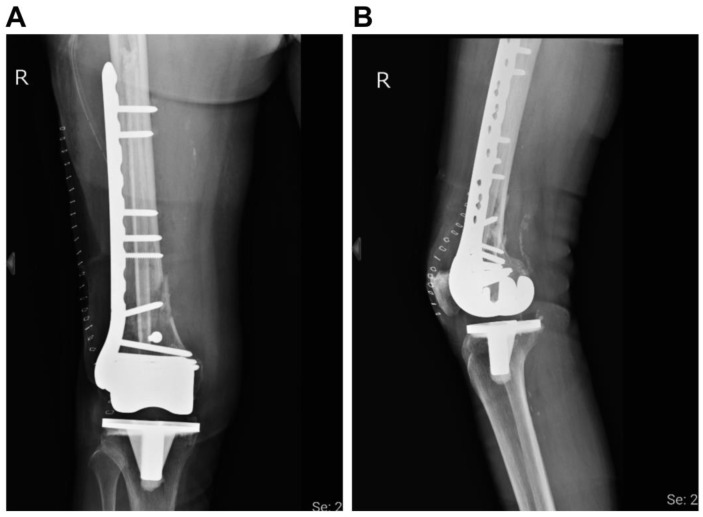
Postoperative radiographic images. (A) Anteroposterior (AP) view of knee. (B) Lateral view of knee. The periprosthetic distal femur fracture is fixed with a locking plate and an allogenous fibula bone graft.
